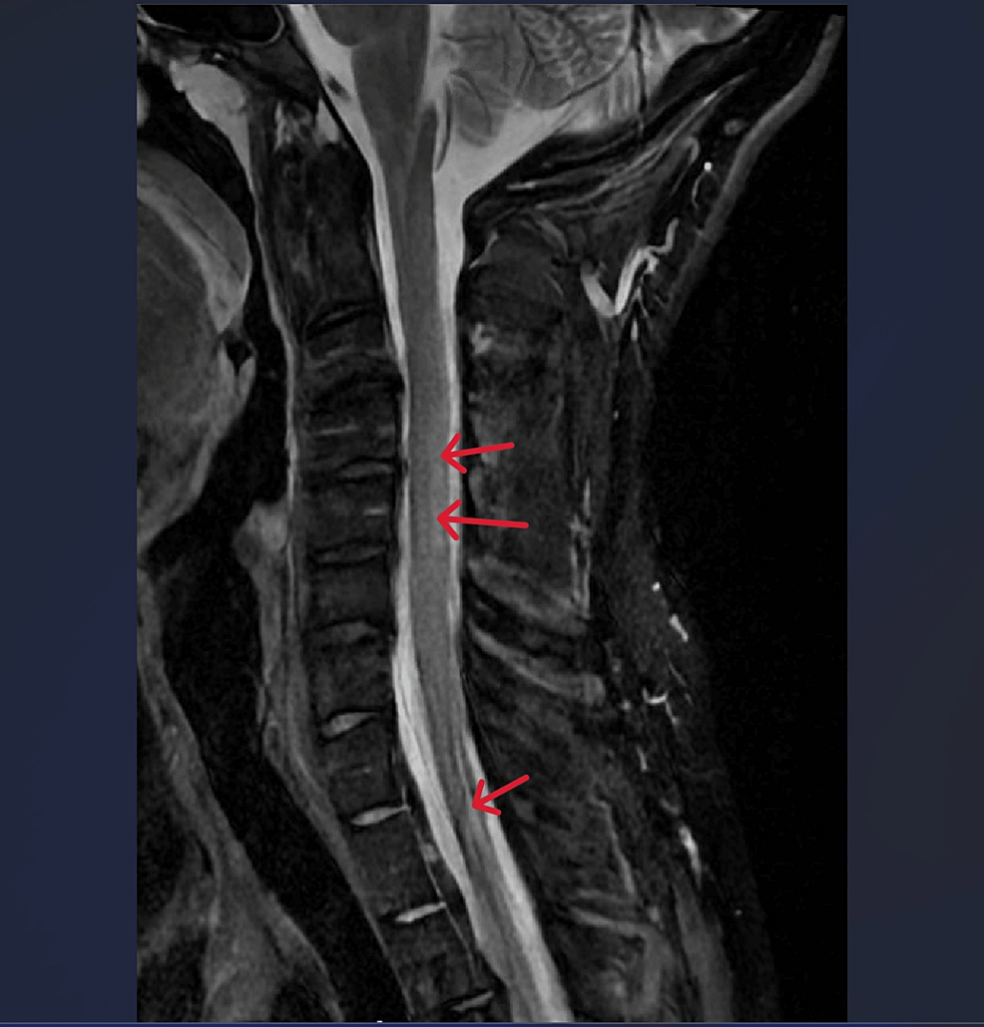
Sagittal MRI cervical spine demonstrating a T2 STIR hyperintense intramedullary signal extending from the level of mid-C2 to the inferior margin of the C7 vertebral body Red arrows point to hyperlucent areas indicative of neurodegeneration.
